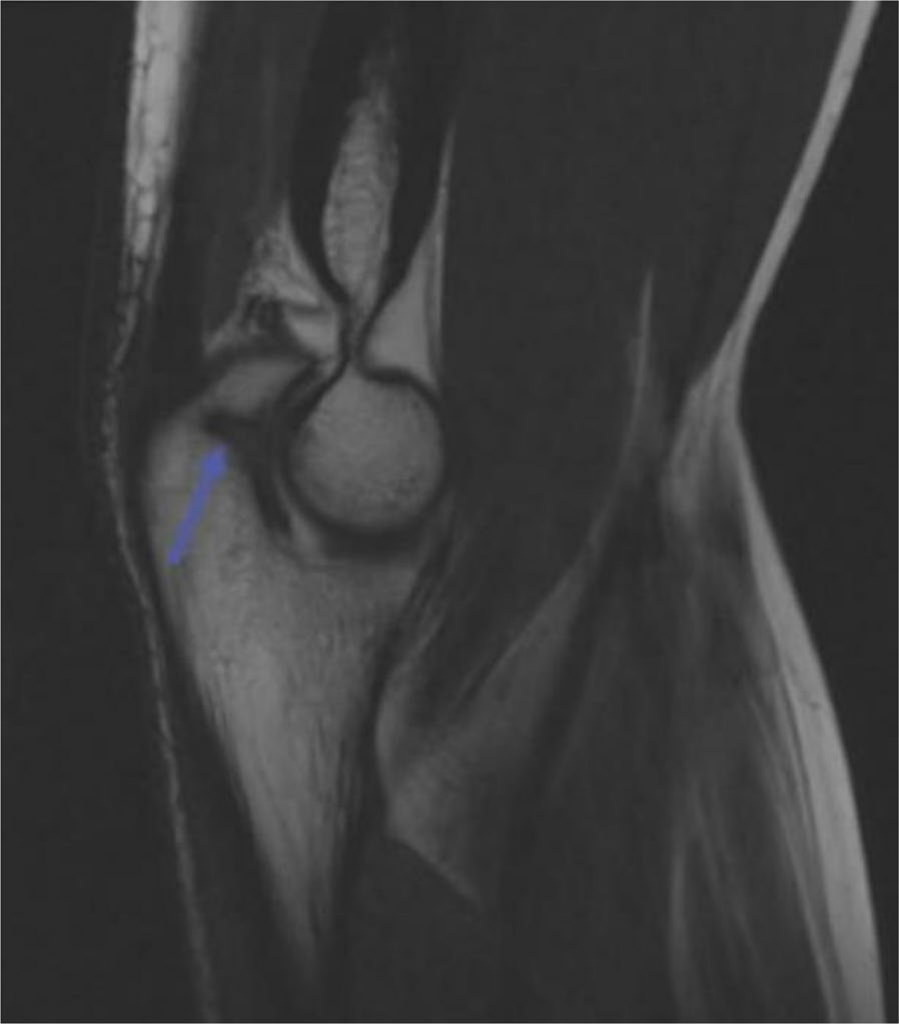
Magnetic resonance image (MRI) demonstrating olecranon stress fracture. Reproduced from Greif et al. [39], with permission
